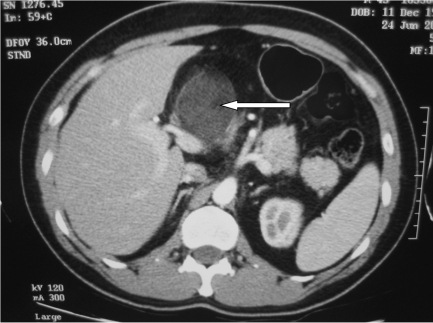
Contrast enhanced CT scan of the abdomen showed a heterogeneous soft tissue mass (white arrow) in the anterior quadrant, lying anterior to the portal vein.
